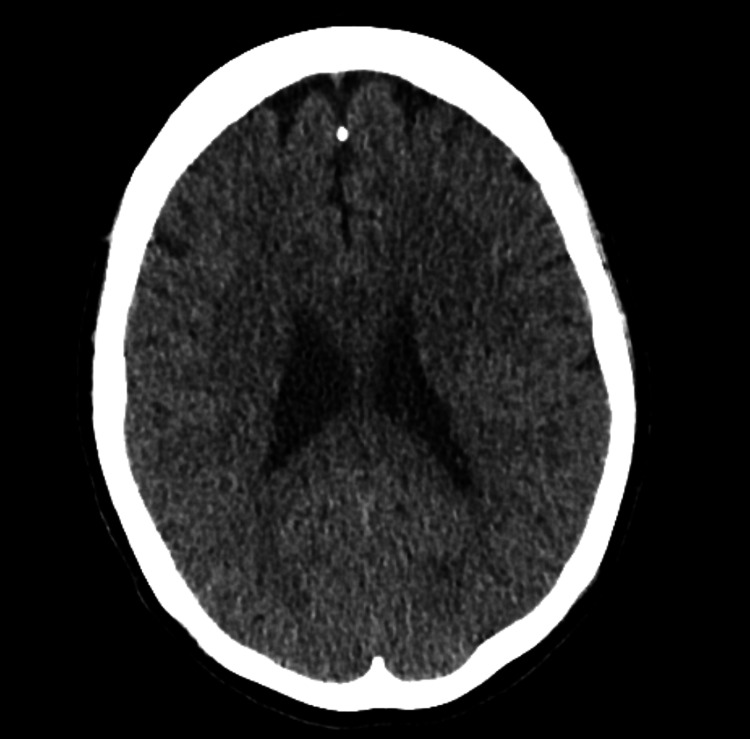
CT head The scan was taken within 12 hours of admission. Impression: No acute findings suggestive of subarachnoid hemorrhage (SAH), infarct, bleeding, or space-occupying lesions (SOL).
